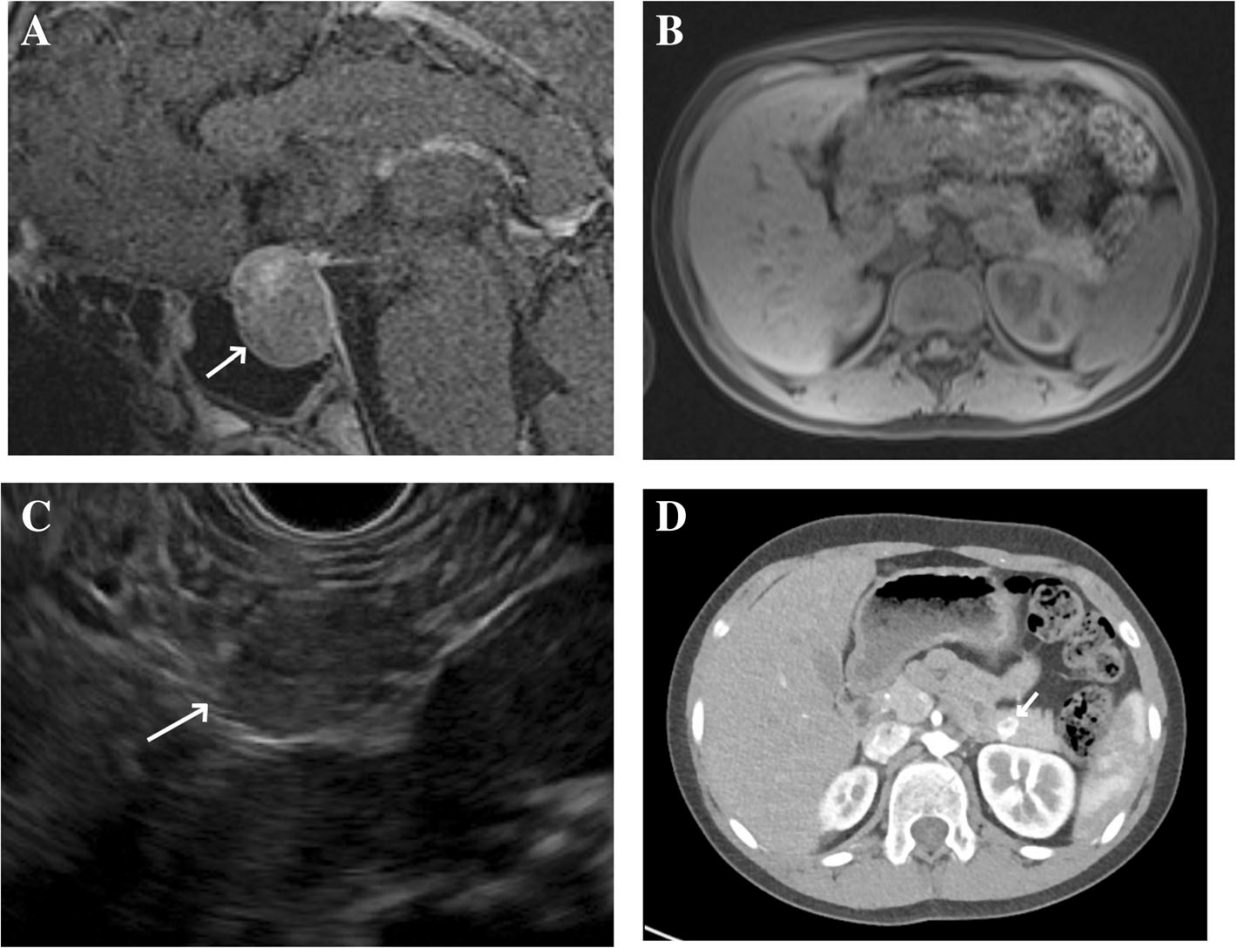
Imaging findings demonstrating pituitary macroadenoma and pancreatic adenoma. a MRI demonstrates enlarged pituitary extending into suprasellar cistern (white arrow) and elevating optic chiasm. b MRI of abdomen demonstrated normal pancreatic signal intensity without identification of any focal lesion or masses. c Endoscopic ultrasound revealed circumscribed hypoechoic mass (white arrow) in body/tail of pancreas. d CT image demonstrating hyperenhancing mass in distal pancreatic body (white arrow)
